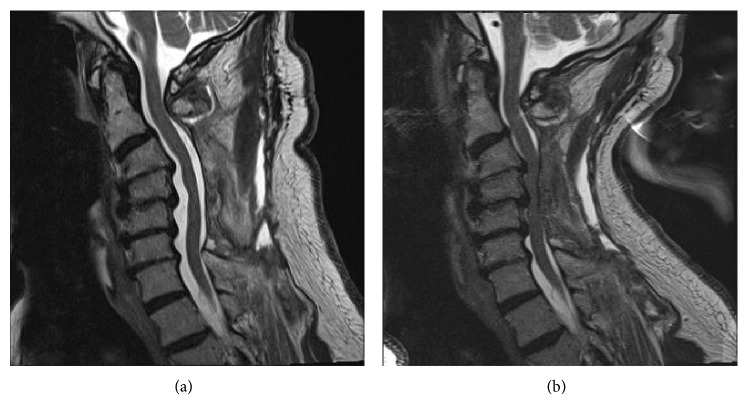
Postoperative T2-weighted sagittal MRI (flexion and extension): spinal cord is compressed by dorsal musculature when the neck is extended. Subaxial spinal alignment (Cobb angle) does not change with flexion-extension, reflecting rigidity of the patient's spondylotic spine.
